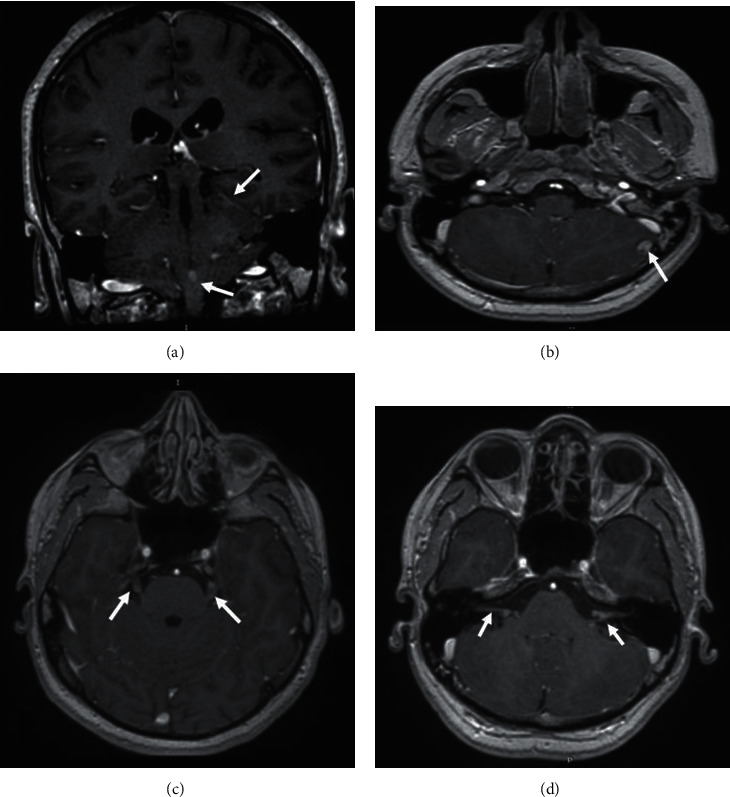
Multisequential multiplanar magnetic resonance images of the brain with contrast. Coronal (a) and axial (b–d) contrasted T1 images reveal multiple subarachnoid nodules slightly enhanced (a, b), bilateral nodular lesions in the trigeminal nerves (c), and acoustic-facial bundles (d).
